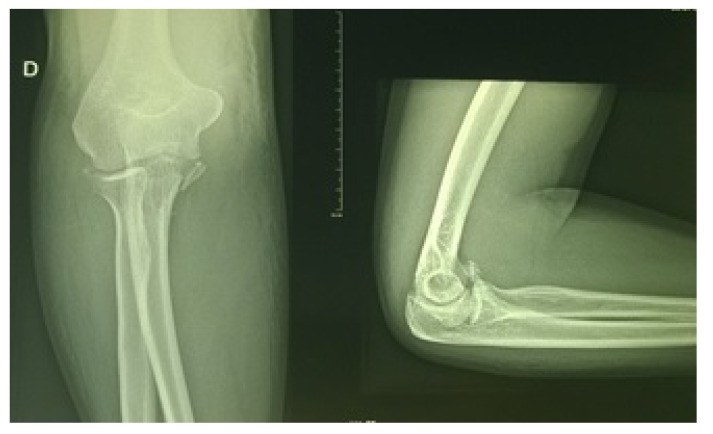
Post-reductional X-ray of the elbow fracture-luxation.
